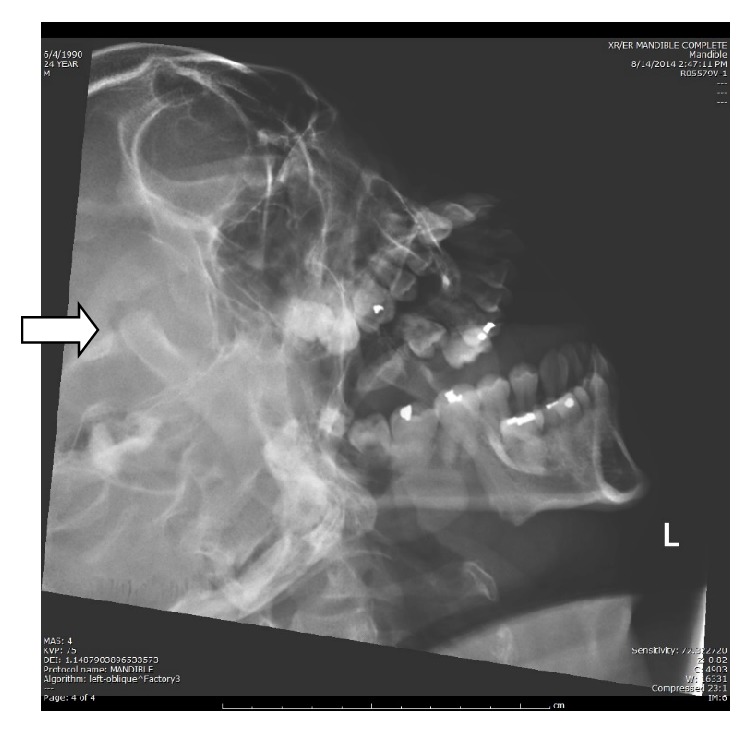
X-ray mandible series (left lateral view). The arrow is pointing to the condylar head.
